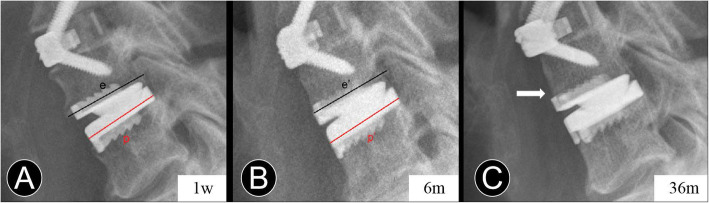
Measurement of anterior bone loss. a and b Immediate and 6-month postoperative lateral radiographs, p and p’ are the length of the prostheses. e and e’ are the length of the endplates. EL% = 100% × (e / p) / (e’ / p’). c At the last follow-up, the lateral radiograph shows peri-prosthesis bone loss at the anterior vertebral body margin (white arrow)
